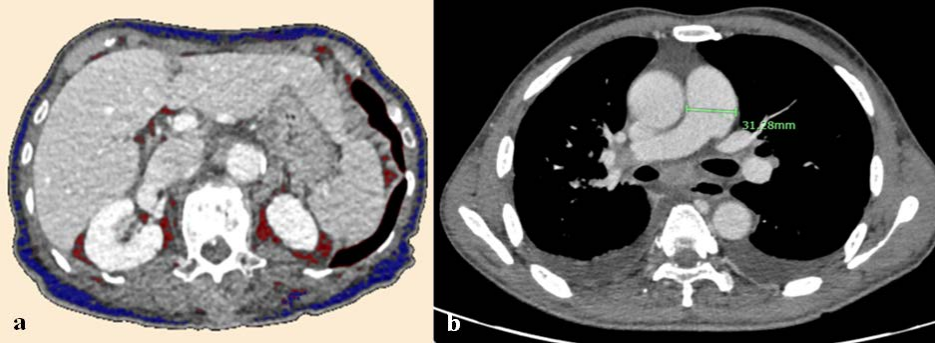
A 54-year-old man with a BMI of 18.03 kg/m^2^ who passed away after 3 months of EGFR-TKI treatment. The axial baseline post-contrast CT scan (a) at L1 level showed SAT = 0.53 cm^2^ (blue label) and VAT = 4.58 cm^2^ (red label) (b) at the level of the pulmonary artery bifurcation showed MPA size = 31.3 mm. BMI; body mass index, EGFR; epidermal growth factor receptor, TKI; tyrosine kinase inhibitor, CT; computed tomography, SAT; subcutaneous adipose tissue, cm; centimeters, VAT; visceral adipose tissue, MPA; main pulmonary artery, mm; millimeters.
